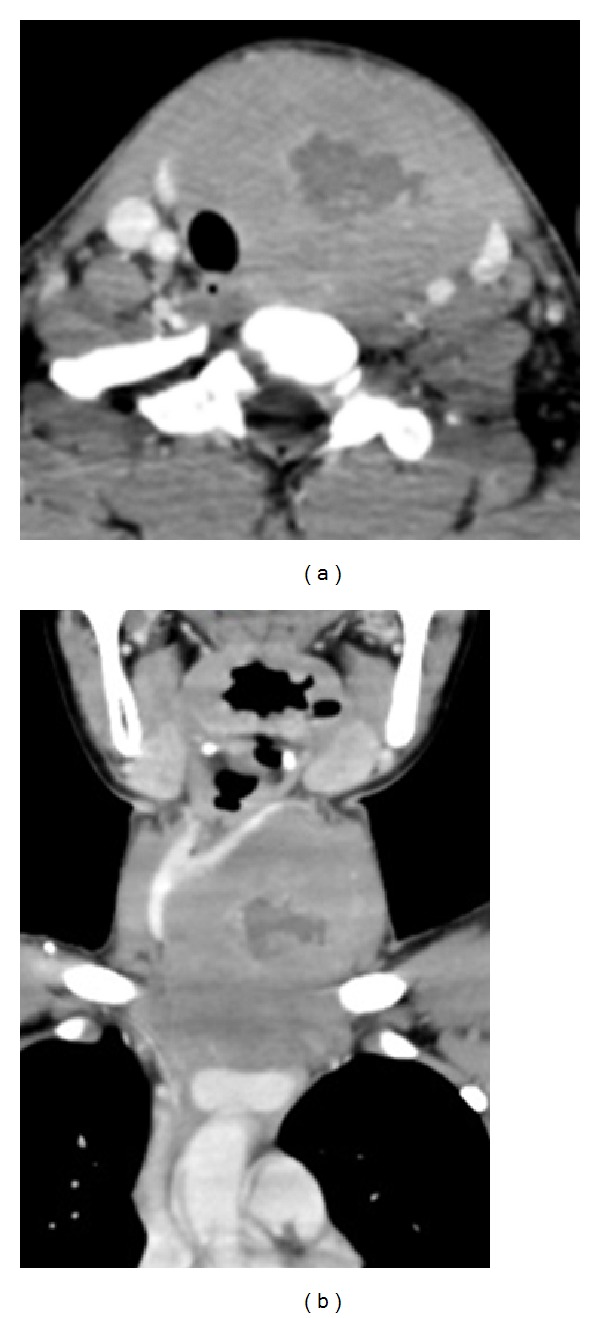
Axial (a) and coronal (b) CT scan showing the thyroid tumor larger than a month before (Figure 2).
